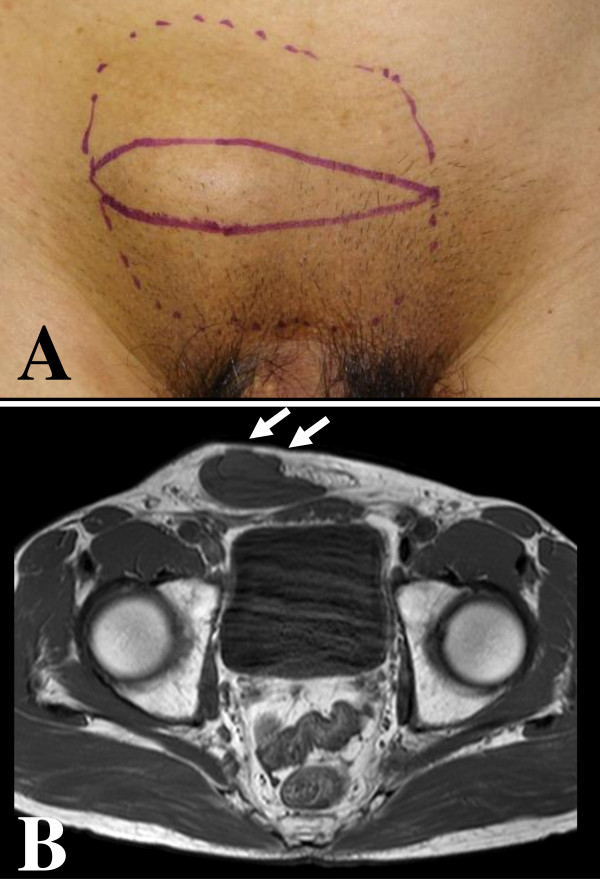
A slowly growing swelling of the soft tissue in the inguinal region. (a) Swelling of the soft tissue in the right inguinal region was observed. (b) Magnetic resonance imaging revealed that the lesion (arrows) was isointense relative to muscle on the T_1_-weighted image.
